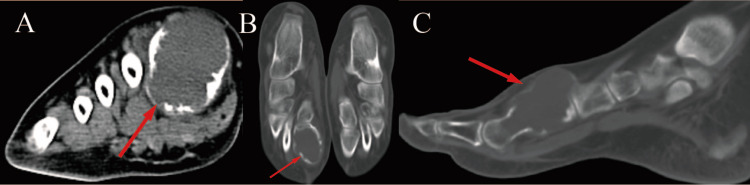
Preoperative CT scan of the feet with multiplanar reconstruction (MPR) Preoperative CT scan of the feet with multiplanar reconstruction (MPR) displays a pathological fracture traversing an expansile osteolytic process within the distal first metatarsal segment, exhibiting cortical expansion, endosteal scalloping, cortical attenuation, and an associated extraosseous soft tissue component.
